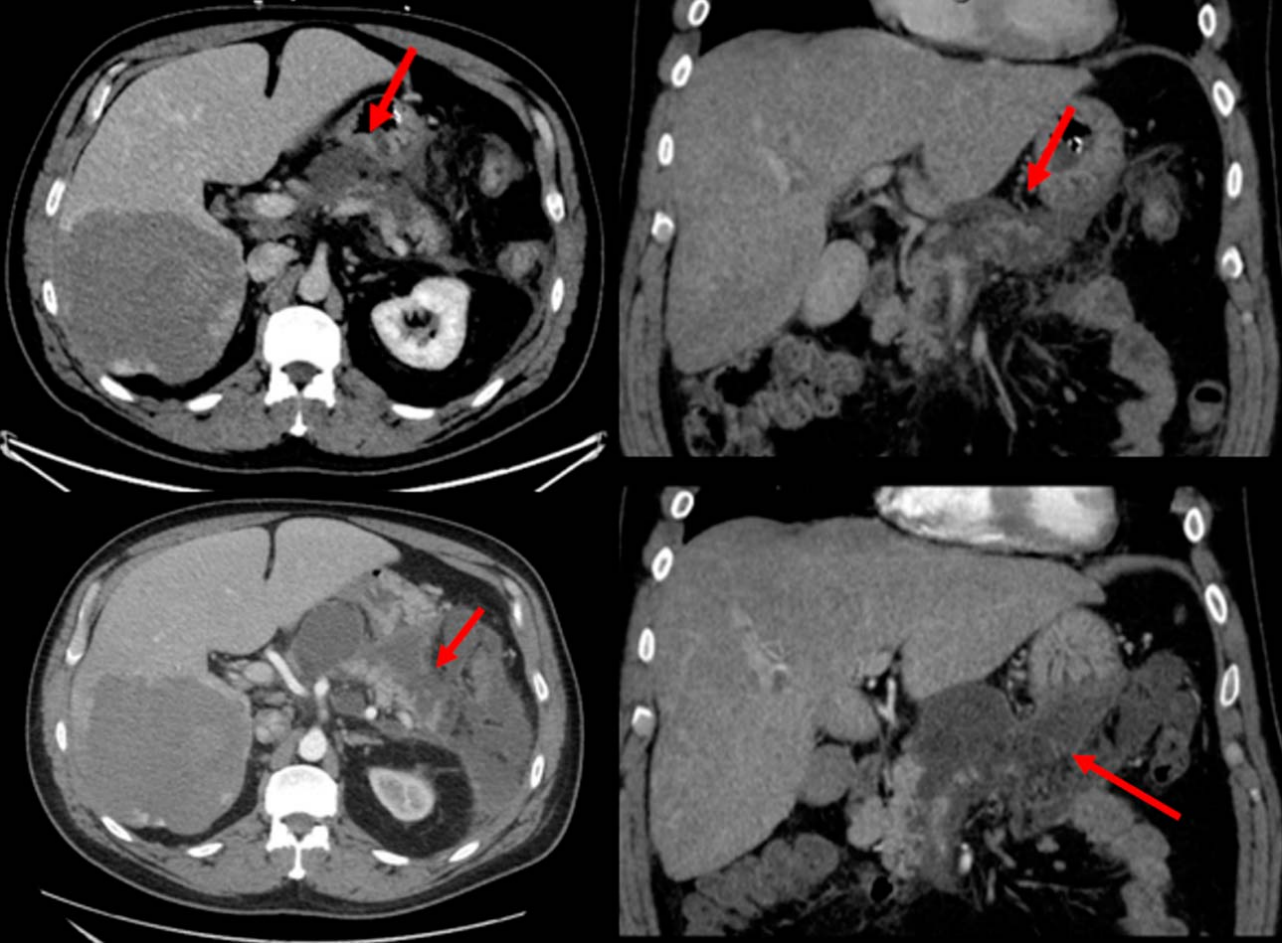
Axial and coronal postcontrast computed tomography scan—two days after liver embolization showing pancreatic necrosis and surrounding edema (arrow) [top image]. Worsening pancreatic necrosis and peripancreatic collections (arrows) 7 days after embolization [bottom image].
